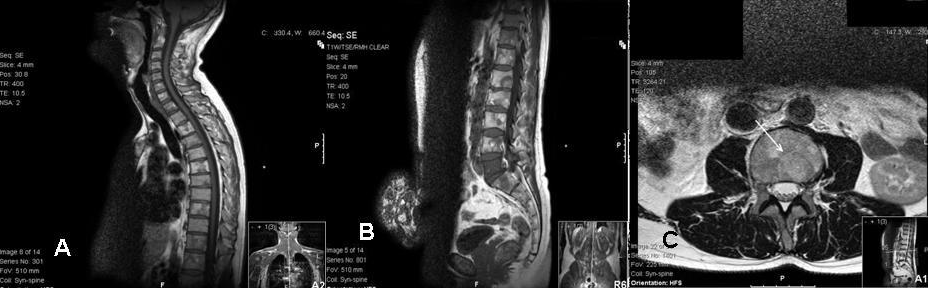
Diffuse abnormal bone marrow signal throughout the spine. Note multiple lesions with high-signal centres on T1W, reflecting the myxoid/fatty nature of the deposits. (a) Sagittal T1W MR iamge of cervical and thoracic spines. (b) Sagittal T1W MR iamge of lumbosacral spine. (c) Transverse section T2W MR image in L3.
